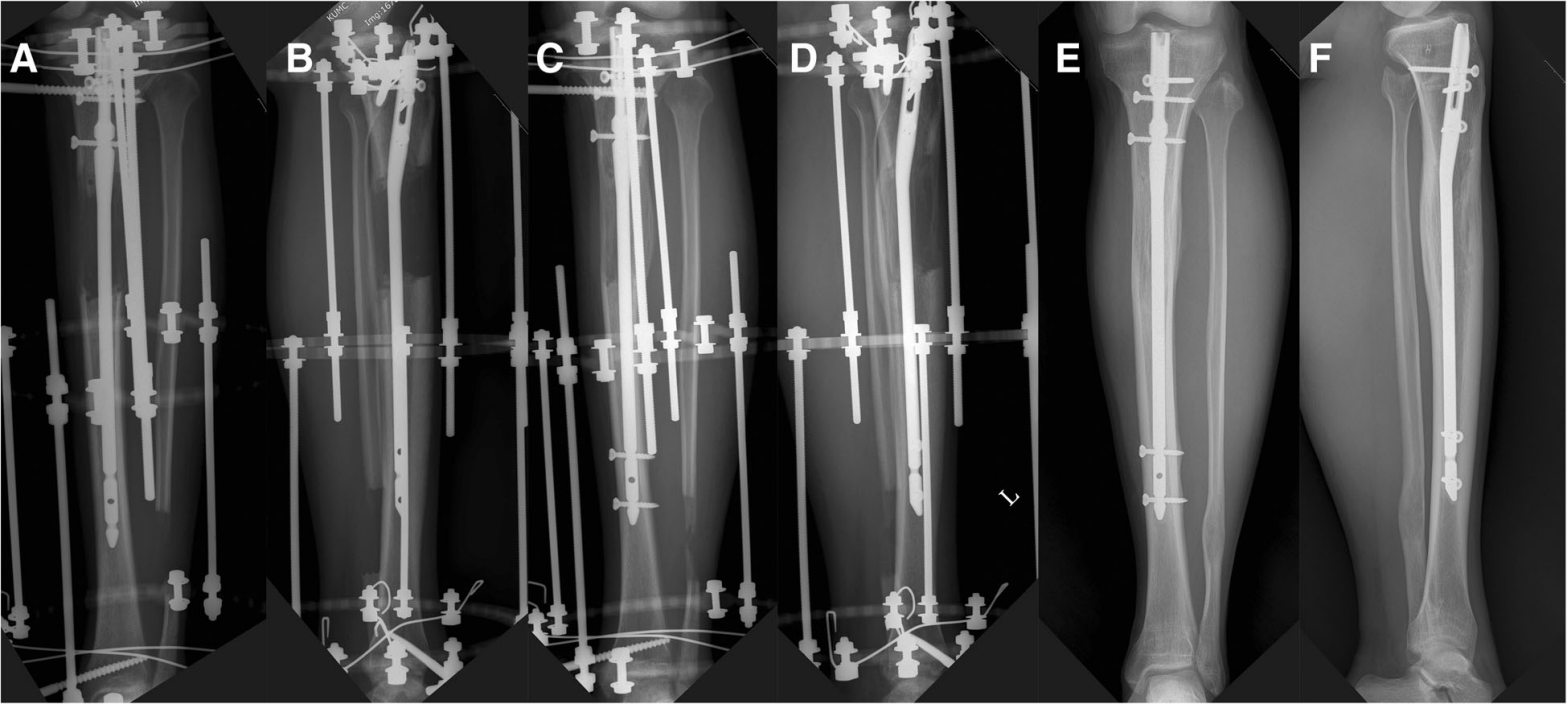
Sequential radiographic images of the left tibia in a 19-year-old female patient without low-intensity pulsed ultrasound (LIPUS) stimulation after 8.1 cm of tibial lengthening. At the end of the distraction phase, the anteroposterior (AP) (a) and lateral (b) images show a callus with a cylindrical shape and low density. At the time of external fixator removal, the AP (c) and lateral (d) images show progression of callus consolidation with a cylindrical shape and intermediate density. The external fixation index of the patient was 29.4 days/cm. At 17 months postoperation, the AP (e) and lateral (f) images show full corticalization of the regenerated callus
